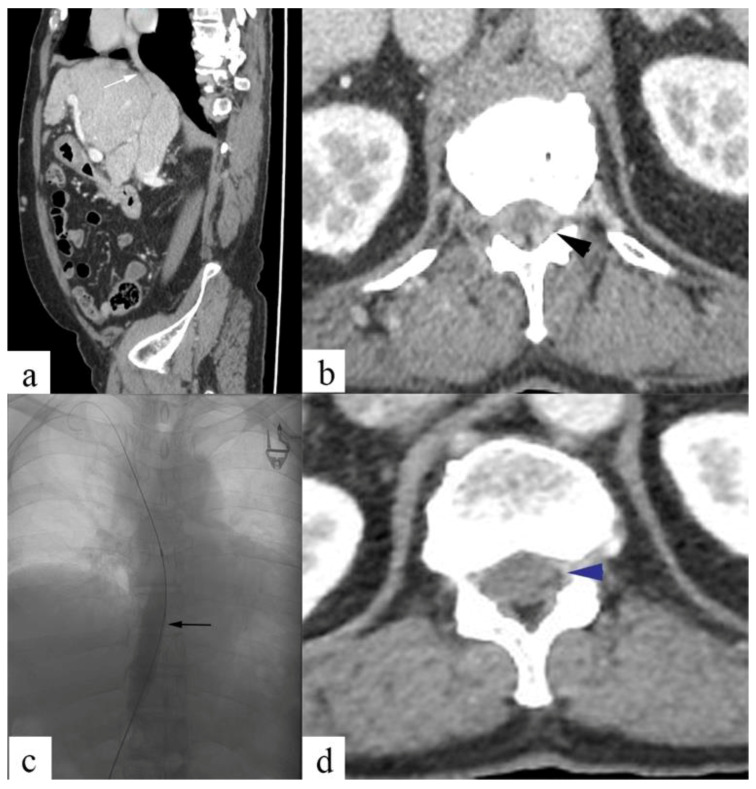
Membranous IVC obstruction in a 29-year-old male patient with Budd–Chiari syndrome and back pain. Contrast-enhanced CT images show membranous IVC obstruction at the suprahepatic IVC (a; white arrow) and epidural venous plexus enlargement (b; black arrowhead). Balloon angioplasty was performed for IVC obstruction (c; black arrow), following which the resolution of EVEs (d; blue arrowhead) was noted, along with back pain relief.
